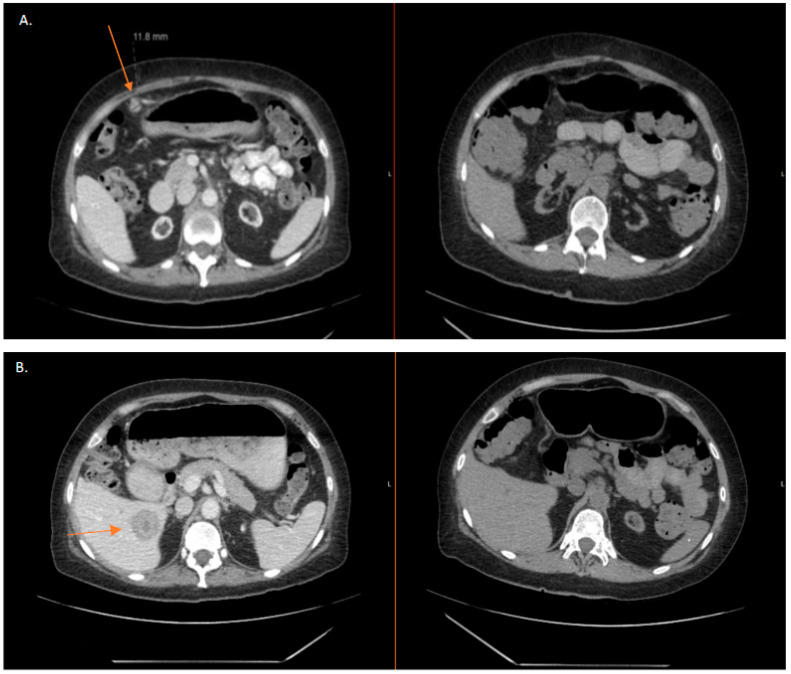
(A,B): CT comparison demonstrating regression of abdoinopelvic metastases represented by arrow. Axial views of metastases in October 2019 compared with corresponding axial views demonstrating complete radiographic regression of abdominopelvic metastases in December 2022.
